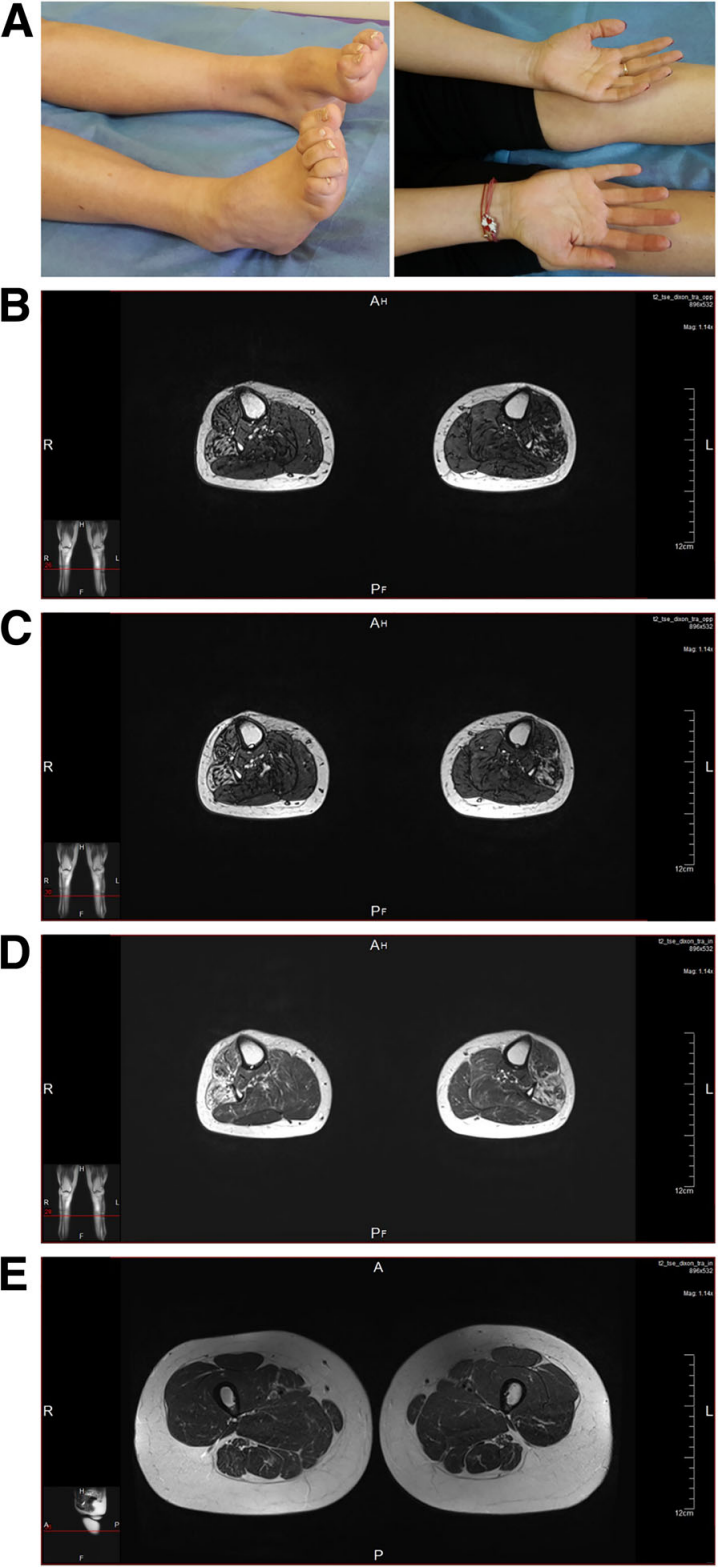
a Clinical features of individual A.III-1, aged 37 years, demonstrating bilateral *pes cavus*, distal hypotrophies in both calves and muscle hypotrophies in the hands, more pronounced for the thenar muscle in comparison with the hypothenar ones. b-e. Magnetic resonance images of individual A.III-1 performed at the age of 37 years. Non-enhanced T1, T2 and proton density (PD) images are shown. b-d. The lateral compartments of the calves, including peroneus longus and brevis muscles are more severely affected, followed by *extensor digitorum brevis* and *tibialis anterior* muscles. The posterior compartment of the calves seems relatively preserved. e At thigh level the muscles are preserved as well, with slight changes visible in semimembranosus and semitendinosus muscles
